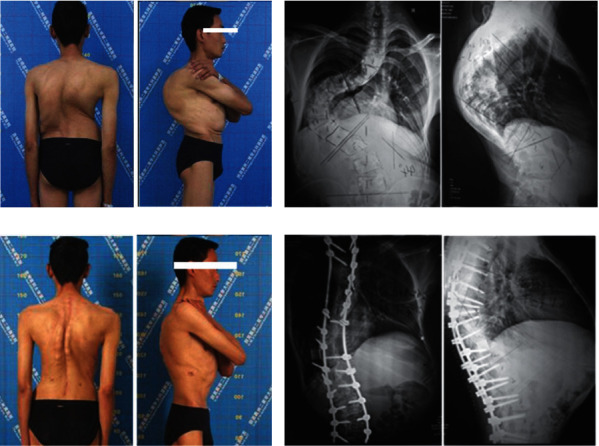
The patient was 35-year-old adult with severe and rigid deformity. (a) Clinical feature preoperative. (b) X-ray of anterior-posterior and lateral preoperative. (c) Clinical feature at 2 years follow-up after PVCR. (d) X-ray at 2 years follow-up.
